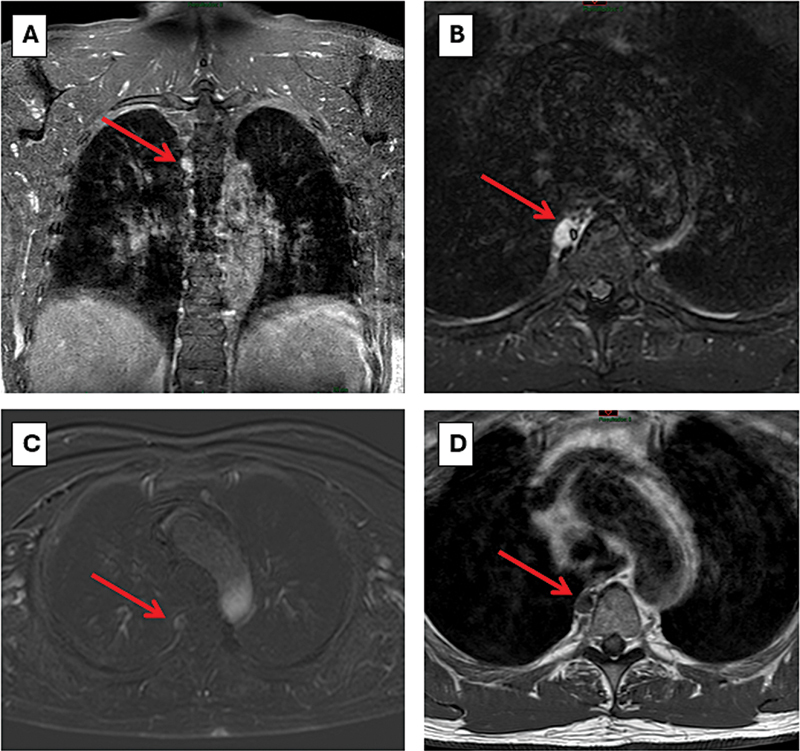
Chest magnetic resonance imaging (MRI) revealed a nodule in the right paravertebral region, at the level of the T4 vertebra (red arrows). It showed contrast enhancement in the portal phase, low signal intensity on T1-weighted images and high signal intensity on T2-weighted images and on the STIR sequence. (
